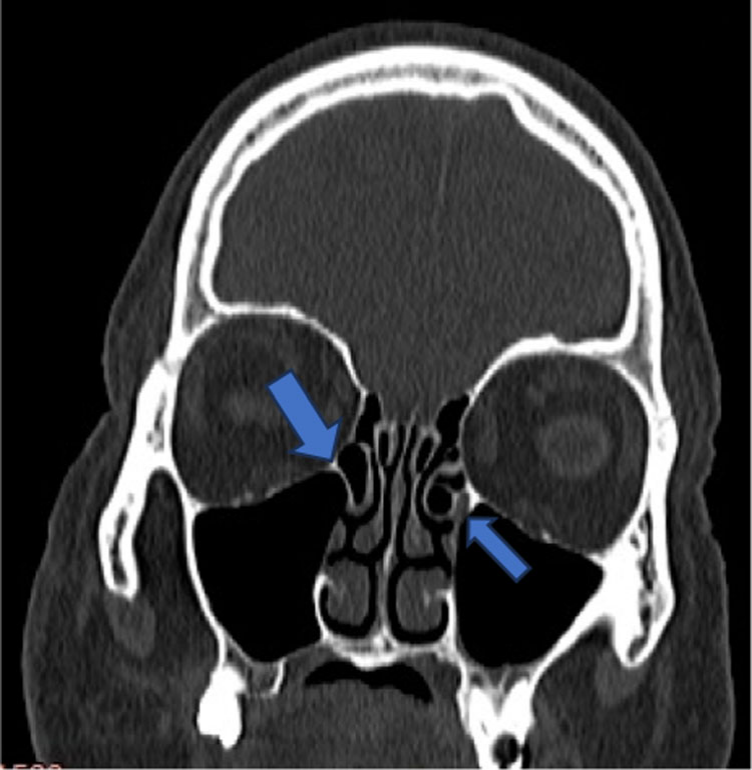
CT coronal image shows ethmoidal bulla or haller cells.
